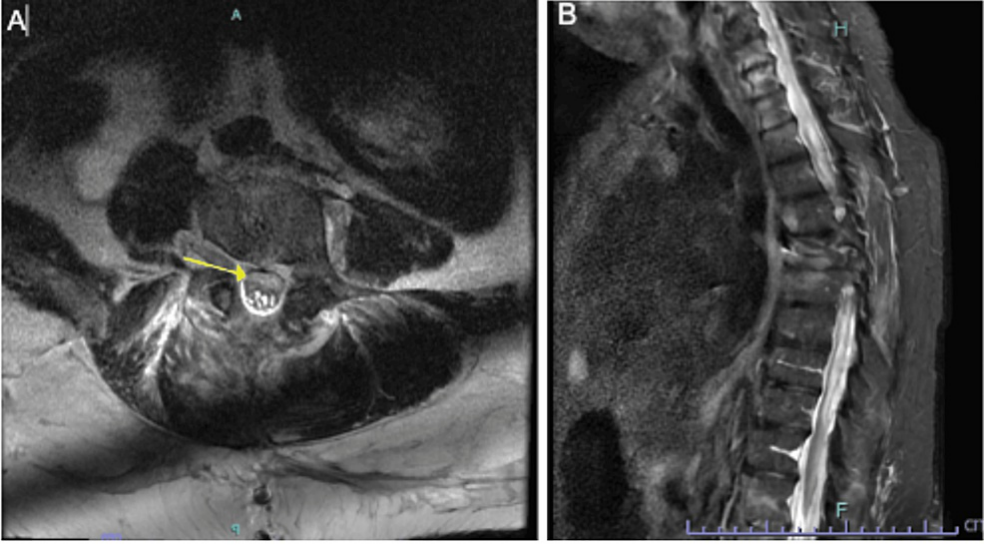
Axial magnetic resonance imaging (MRI) without contrast of the lumbar spine Figure 2A (left) denotes the clumping of the nerve roots posterior to the collection, as seen in the T2 axial image at L4. The yellow arrow denotes an area of phlegmon that is distinct from CSF and clumped roots in the spinal canal. Figure 2B (right) captures a sagittal T2 view that demonstrates the multilevel edema of the spinal cord. CSF: cerebrospinal fluid
